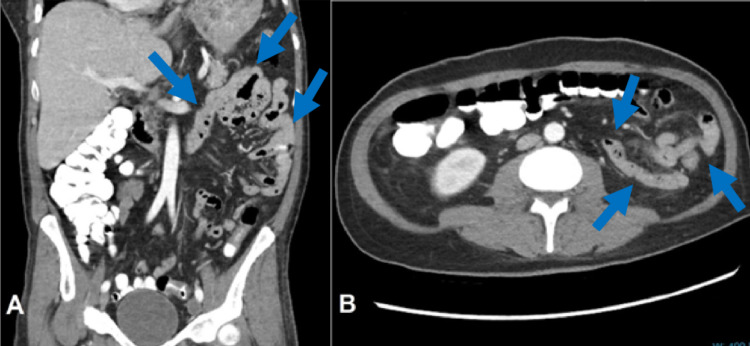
CT of the abdomen and pelvis with contrast revealing diffuse bowel thickening of the descending and sigmoid colon as well as the rectum in coronal (A) and axial (B) sections (blue arrows).
